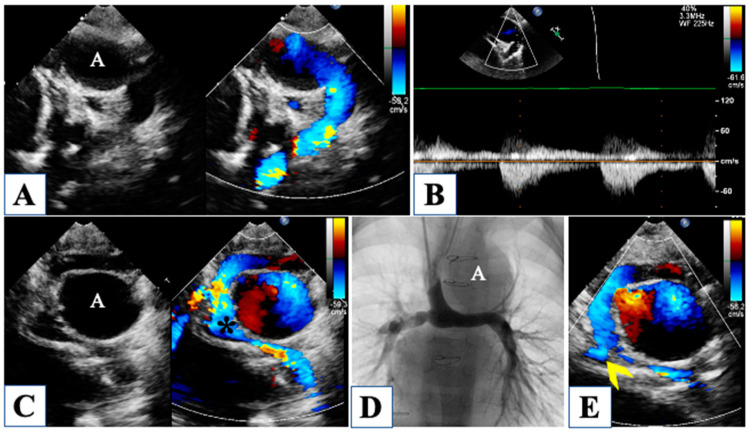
Prominent aortic arch reconstruction with dilatation of the transverse arch (A), compared to the normal size of the descending aorta, without Doppler signs of obstruction (Panel (A,B)). In panel (C), the superior cavo-pulmonary anastomosis (*) with partial compression of the pulmonary branches. Angiography of the pulmonary branches (Panel (D)) shows significant stenosis of the right pulmonary artery, whose ostium (arrowhead) is not easily visualized by echocardiography (Panel (E)).
